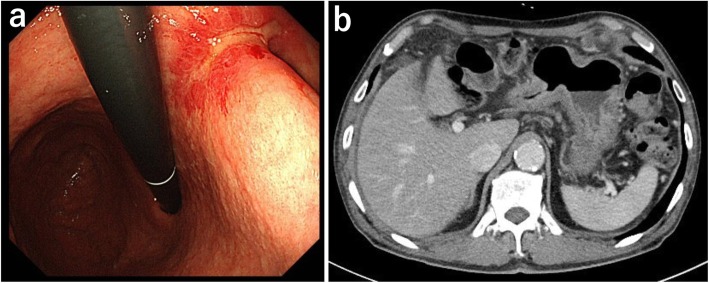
Imaging after combined therapy. a Gastroscopy showed only a small ulcer in the area of the original tumor. b Computed tomography scan showed reduced thickening of the gastric wall and marked shrinkage of regional lymph nodes
